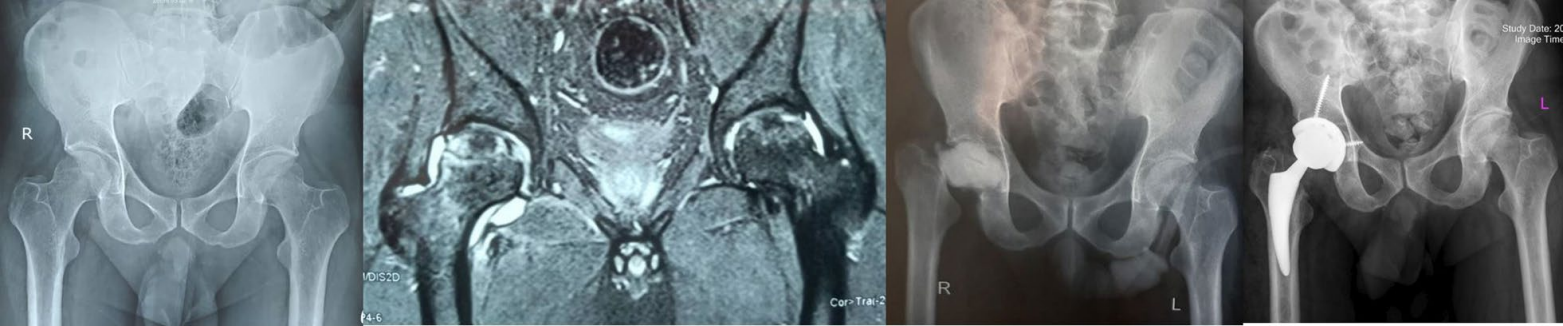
a Case five, preoperative X-ray, both sides involvement. b MRI of the hips shows right side cartilage destruction and less involvement of the left side. c Post-op radiography after first stage of right side and arthrotomy and irrigation and debridement of left side. d Final radiography after second stage THA of right side
